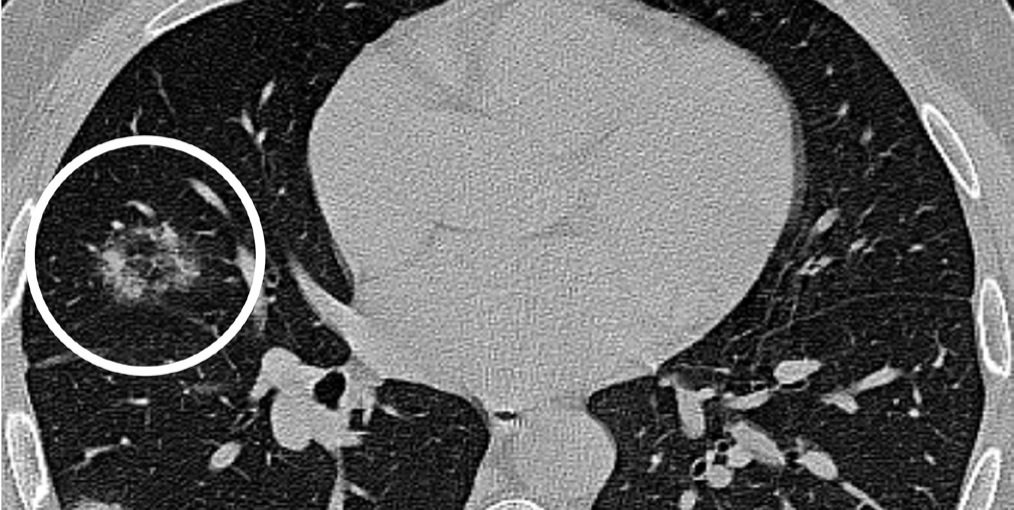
A 32-year-old female infected with COVID-19 (δ Variant): axial unenhanced CT image showed focus of central ground glass nodule surrounded by an inner ring of air and an outer ring of ground glass (white circle). This appearance ressembles a bullseye.
